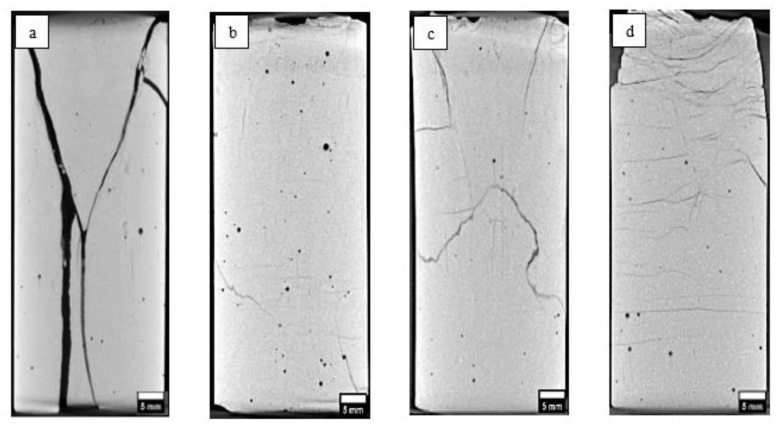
CT scans of cement cores after failure under triaxial testing at a confining pressure of 13.7 MPa and 90 °C: (a) neat Class-H cement showing the failure in comparison to the others; (b) 5% ferrierite-added cement; (c) 15% ferrierite-added cement; (d) 30% ferrierite-added cement.
